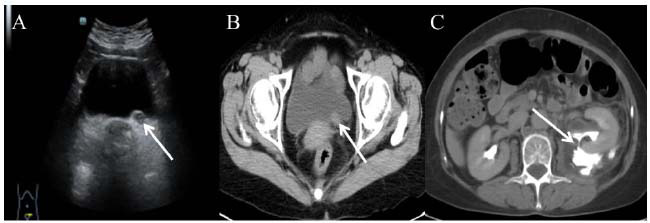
Ultrasound and CT scan of the lesion. Trans-abdominal ultrasound showed a hypoechoic lesion in the left distal ureter (A, arrow). The CT scan showed a lesion (B, arrow), accompanied by left hydronephrosis and rupture of the renal pelvis (C, arrow).
